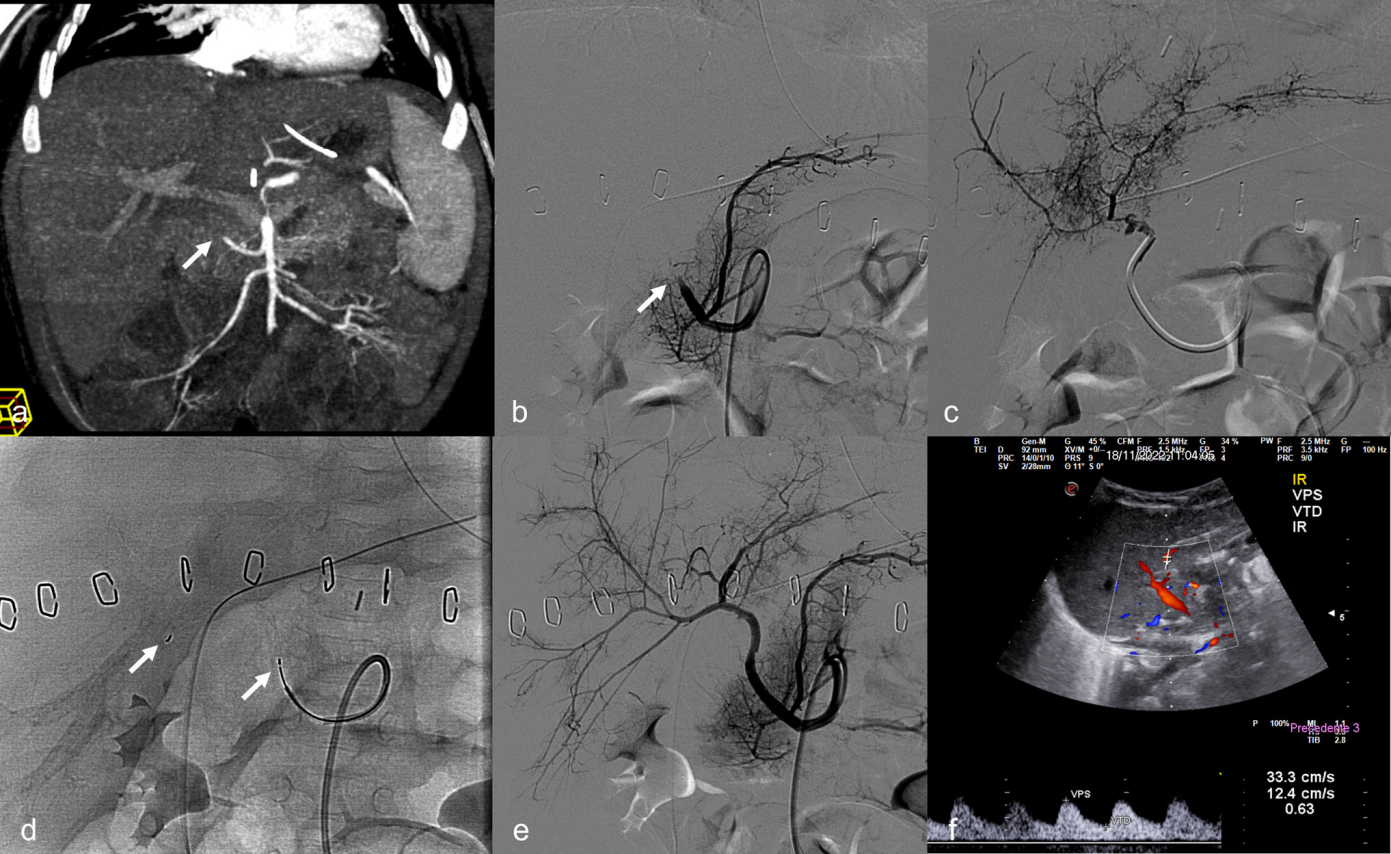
Acute hepatic artery thrombosis in a 8-month-old female (case #10) 7 days after whole liver transplantation. a CT-angiography coronal MIP shows abrupt occlusion of the hepatic artery (arrow) originating from the superior mesenteric artery; b selective angiogram of the hepatic artery confirms the occlusion at the surgical anastomosis (arrow); c selective angiogram performed after recanalization of the hepatic artery with a 4-F catheter shows a very poor intrahepatic arterial circulation; d X-ray image acquired during stent-retriever thrombectomy: the arrows indicate the markers at the extremities of the Solitaire AB device; e final angiogram of the hepatic artery shows restored opacification of the hepatic artery and its main intrahepatic branches. d Follow-up color-Doppler ultrasound shows the patency of the hepatic artery with a normal waveform
